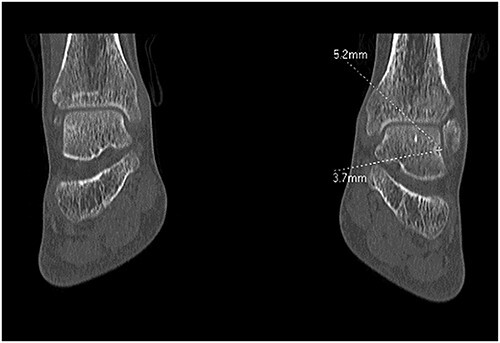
CT right ankle, arrow osteochondral lesion of the talus.
